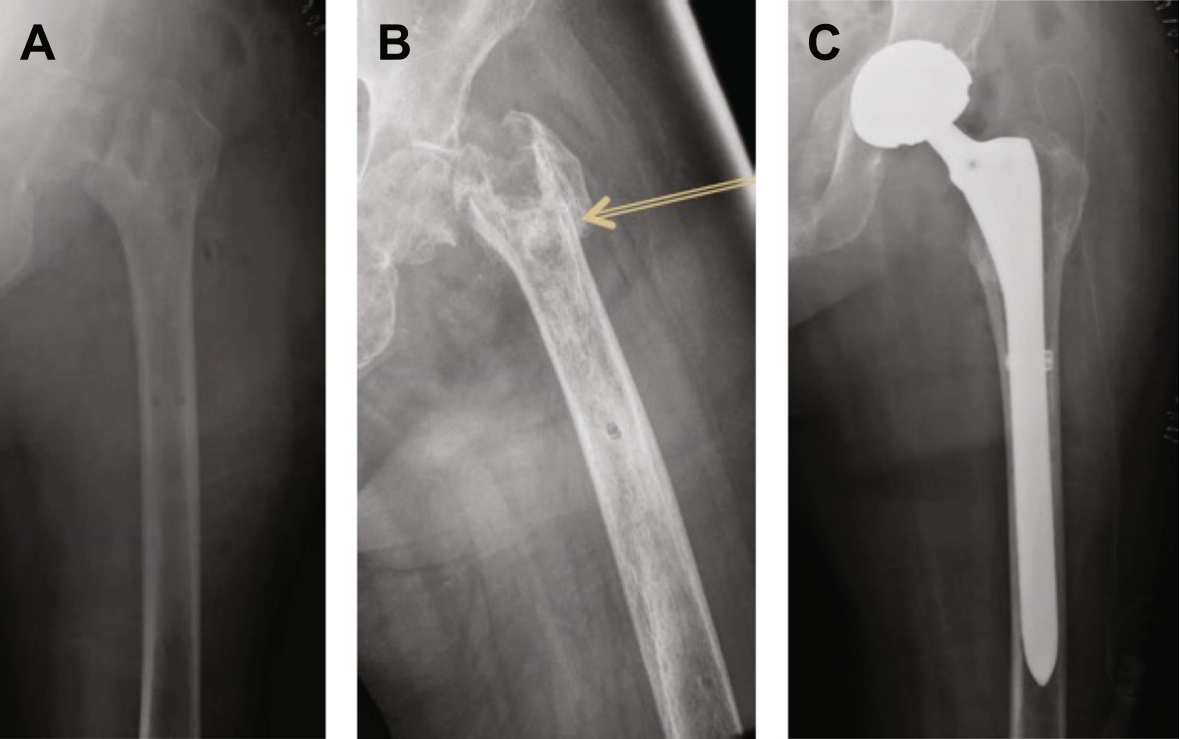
Post-operative radiographs of the 2-stage THA procedure in patient #2. a Post-operative radiograph for Stage 1 of THA. The fixation hardware was removed and the void was filled with V-CBA to rebuild the bone stock. b Radiograph 2 months after placing V-CBA showed some consolidation of the bone graft is evident (yellow arrow). c Post-operative radiograph for Stage 2 of THA. The second procedure was performed 3 months after V-CBA was implanted
